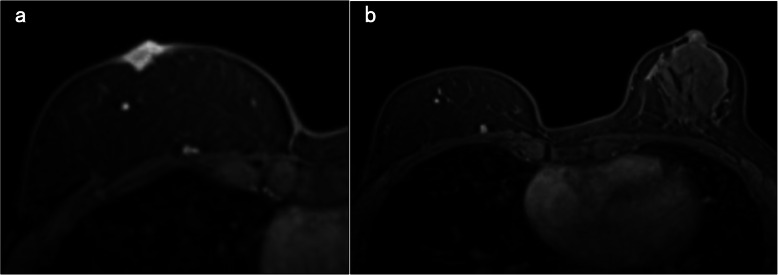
a Magnetic resonance imaging (MRI) shows enhancement of the lesion of the right nipple and slight subcutaneous adipose tissue. b There is no abnormal enhancement in the left breast
